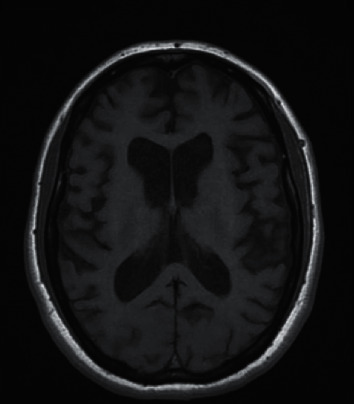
Axial T1-weighted MRI of the brain was performed on a 1.5 Tesla scanner showing generalized moderate cortical atrophy for the patient's age.
